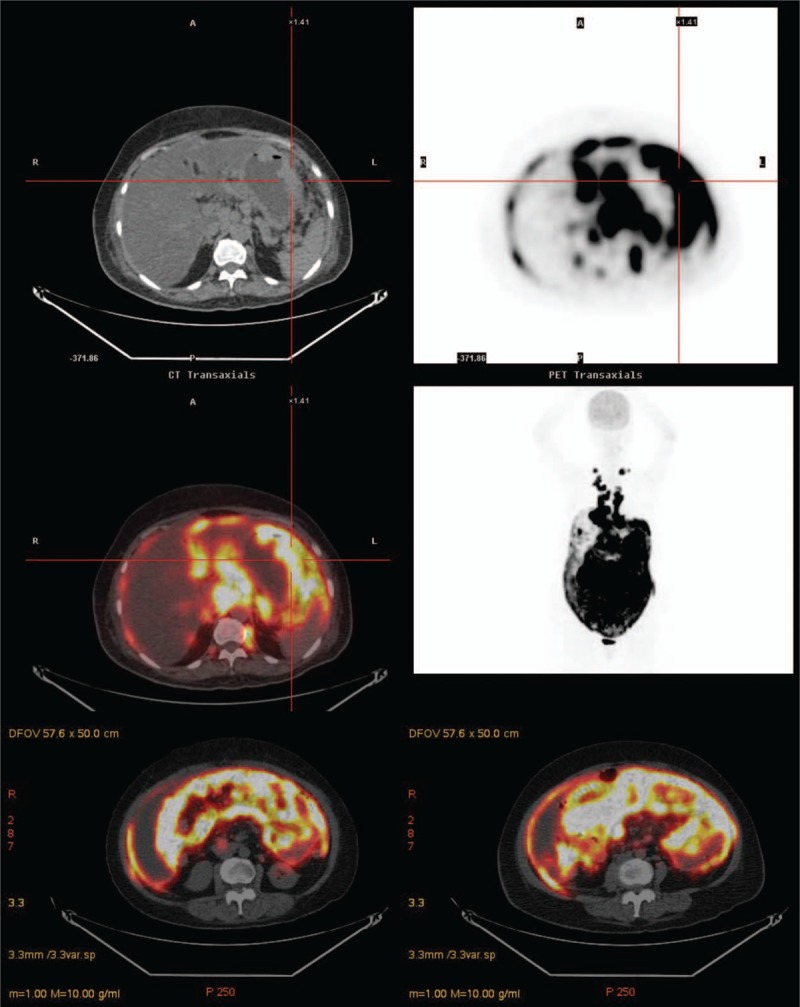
PET scan showing intense FDG uptake in the omentum and stomach corresponding to peritoneal lymphomatosis and stomach invasion. PET = positron emission tomography, FDG = fluorodeoxyglucose.
